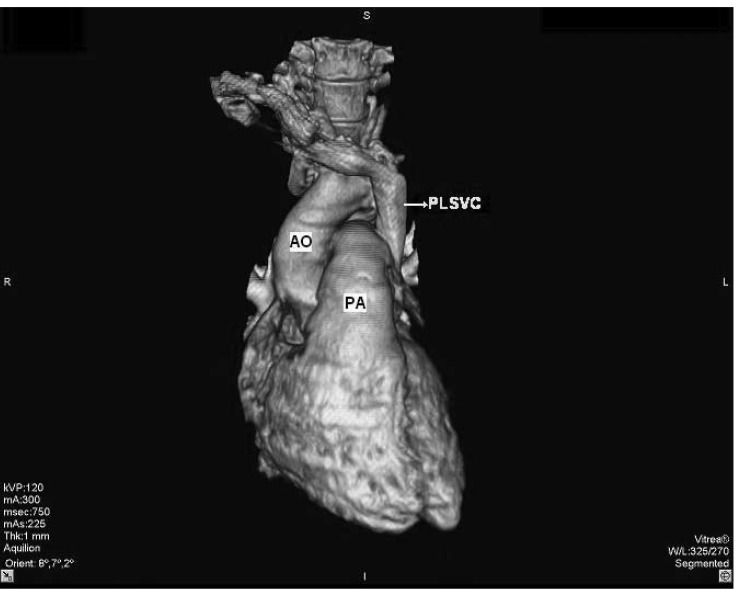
Volume-rendered, three-dimensional reconstruction of contrast-enhanced multi-detector computed tomographic image shows the persistent left superior vena cava (PLSV C) descending on the left side of the thorax (AO: aorta, PA: pulmonary artery).
